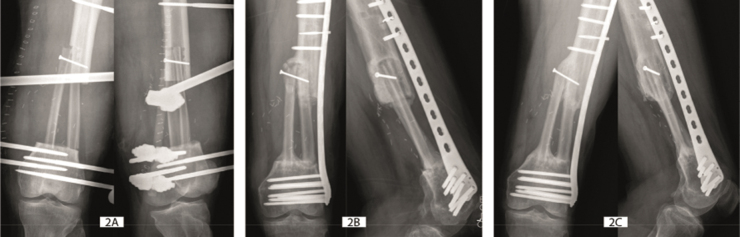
A. Double-barrel femoral reconstruction following resection of an osteomyelitic segment. B. Following an initial external fixation period of 6 weeks, stability was achieved with a permanent bridging plate. C. X-rays taken during the 27^th^ month after the initial operation reveal limited hypertrophy (type 1) due to stress shielding of the strong plate.
